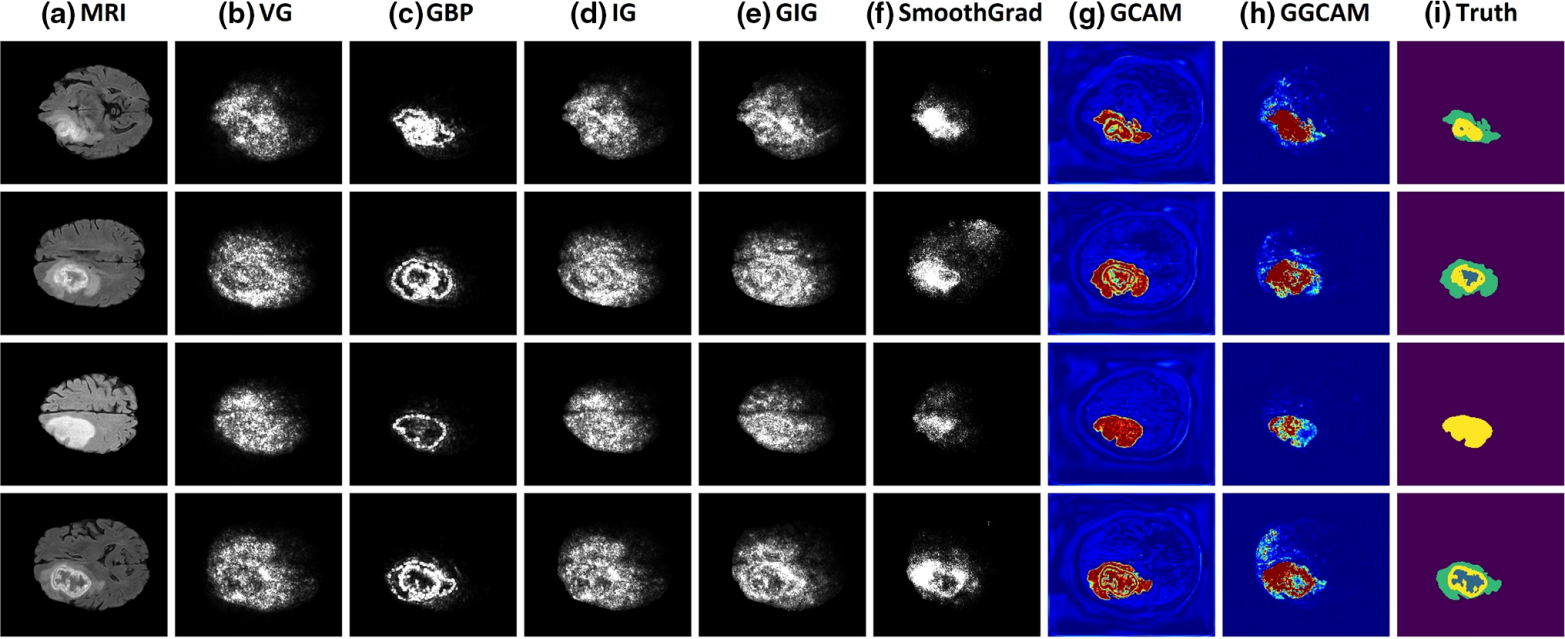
Comparing different XAI visualization methods for brain glioma segmentation. Left to right: original MRI image, Vanilla gradient, guided backpropagation, integrated gradients, guided integrated gradients, SmoothGrad, Grad-CAM, guided Grad-CAM, and the manual truth annotations. Note that in (b, c, d, e, f), all contributing features are highlighted in white, while in (g, h), red regions correspond to a high score for the tumor region
